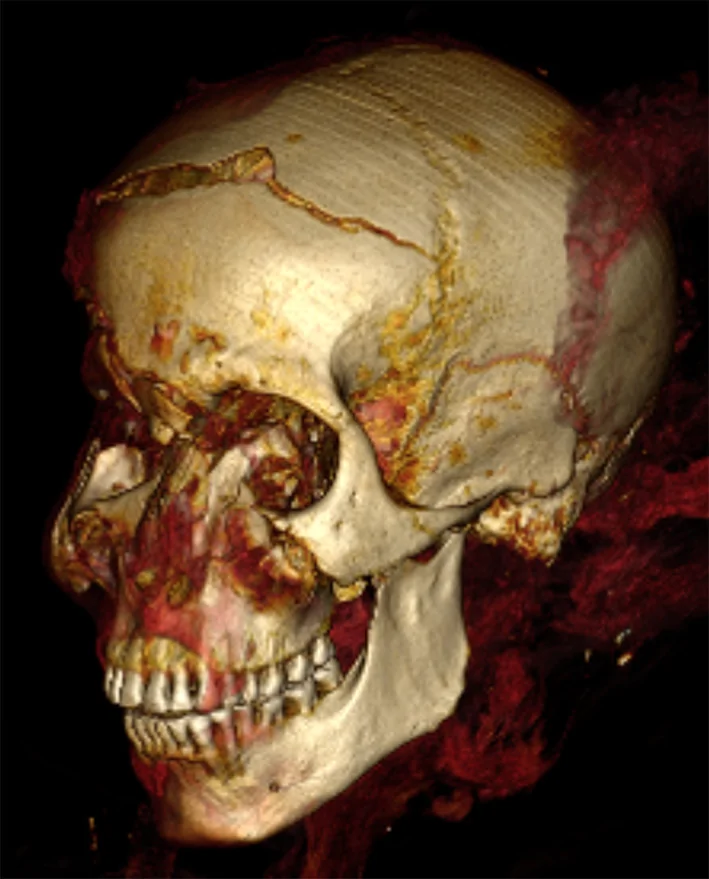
Three-Dimensional CT image of Seqenenre's head in left oblique projection shows an oblique cut wound of the left zygoma, fracture of left coronoid process of the mandible.
